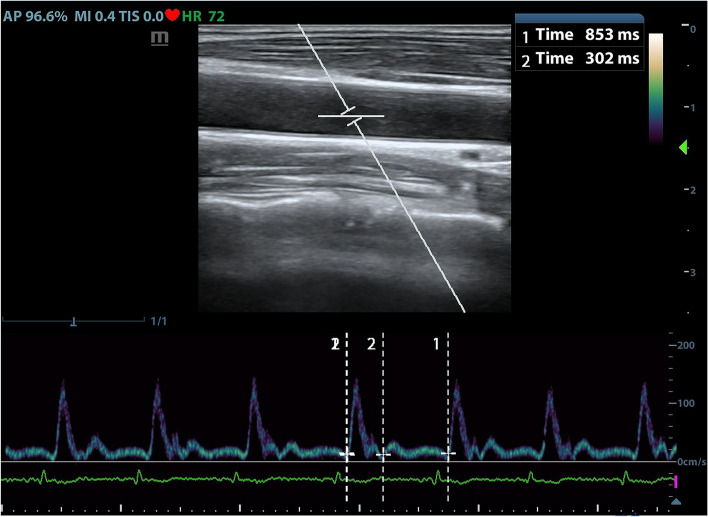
Ultrasound measurements of the carotid artery and calculation of FTc. The panel above shows a two-dimensional scan of the right common carotid artery, and the following panel shows the corresponding blood flow spectrum. “1 Time” indicates the systolic flow time (ST), and “2 Time” presents the cycle time (CT)
